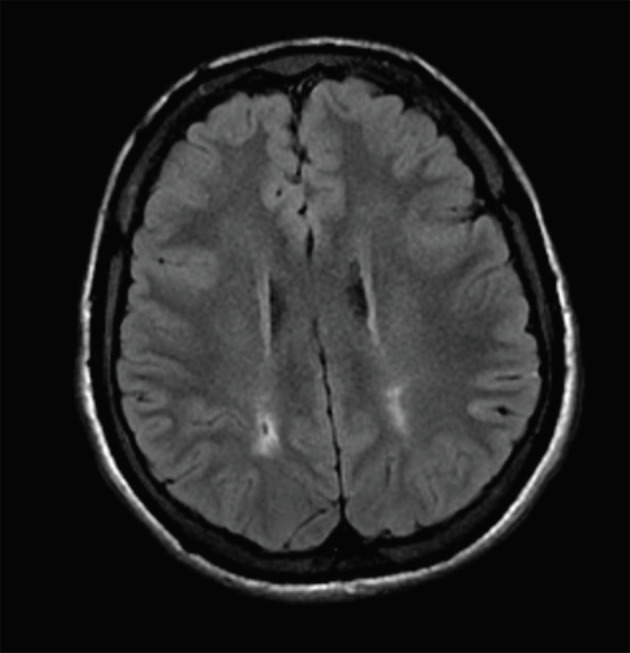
Axial FLAIR MR image. Biparietal white matter hyperintensities with cystic changes at right.
